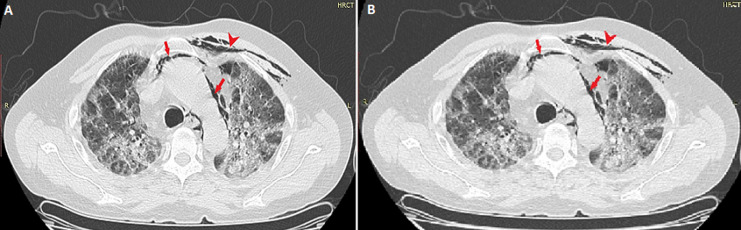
A, B) axial CT chest showing multifocal, bilateral ground glass opacities, predominantly in peripheral region and posterior lobes. Pneumomediastinum seen around aorta (arrows) and left paratracheal region. Subcutaneous emphysema is also visible (arrow head)
